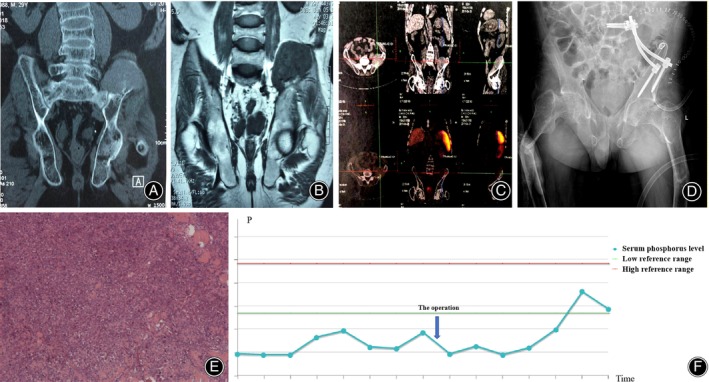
Case 2 (A) CT of the hip showing the suspected culprit tumor of the left acetabulum. (B) MRI of the hip revealing the suspected causative tumor. (C) The first‐line ^68^Ga‐DOTATATE PET/CT scan showing high intake in the left acetabulum. (D) Postoperative X‐ray demonstrating the position of the internal fixation was satisfactory. (E) Pathological results confirmed the diagnosis of phosphaturic mesenchymal tumor. (F) Serum phosphorus levels elevated to the normal range postoperative.
